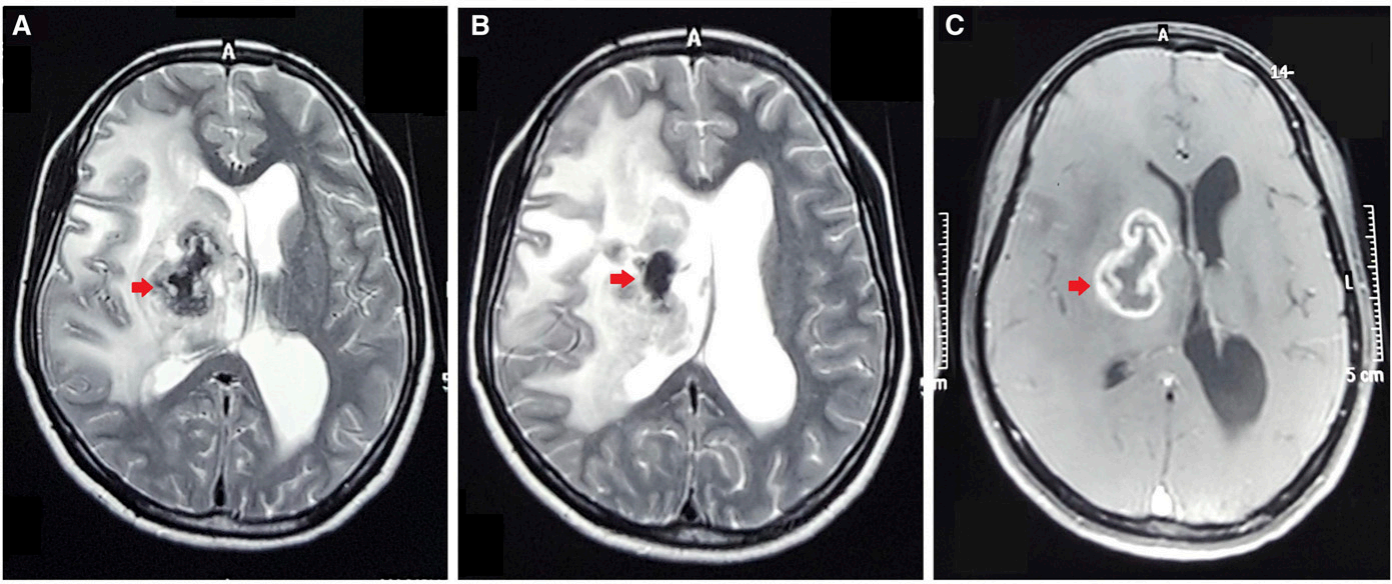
(A, B) Tuberculoma in the right basal ganglia with markedly hypointense center (arrow) in T2-weighted magnetic resonance imaging and (C) ring enhancement on contrast administration. This figure appears in color at www.ajtmh.org.
